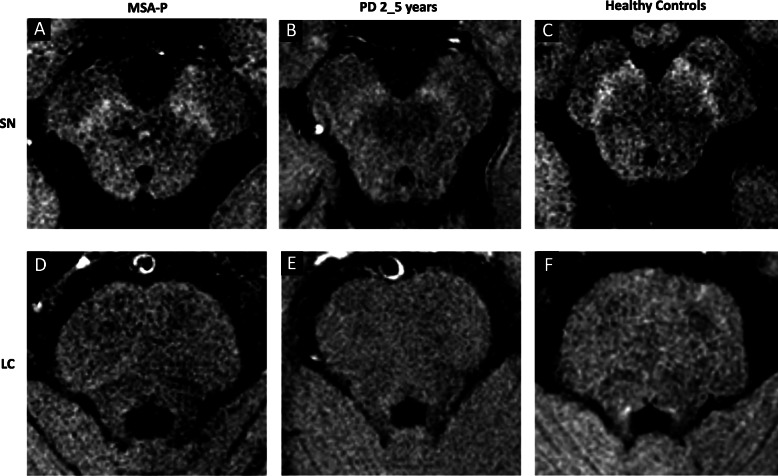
Neuromelanin-sensitive MRI axial images of SN (a-c) and LC (d-f) in a patient with MSA-P (a, d), PD with 2–5 years of duration (b, e) and a healthy control (c, f)
